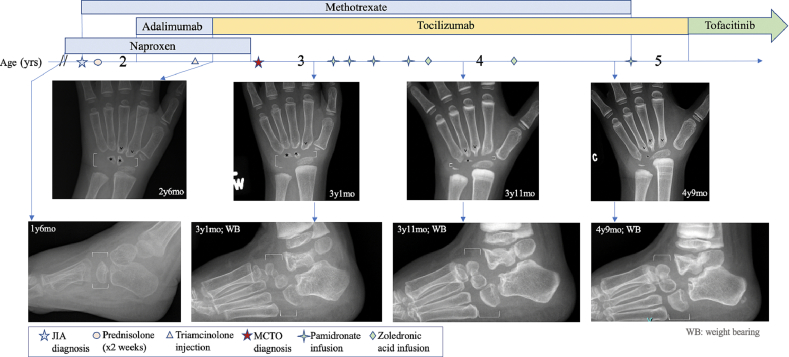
Left wrist and right ankle films from age 1 year 6 months to 4 years 9 months. Radiographs of the patient's wrist and ankle in relation to treatments received show progressive osteolysis and atypical bone formation. The joint compartments (represented by brackets) are narrow and hypoplastic with significantly decreased volume within the joint space. There are fewer carpal bones than expected for age, and the ones that are present (asterisks) appear qualitatively smaller and dystrophic from an early age. Increased erosions of the proximal metacarpals (v) are observed during treatment. The weight bearing bones of the mid- and hind-foot appear stable to slightly increased in size after initiating bisphosphonate treatment.
